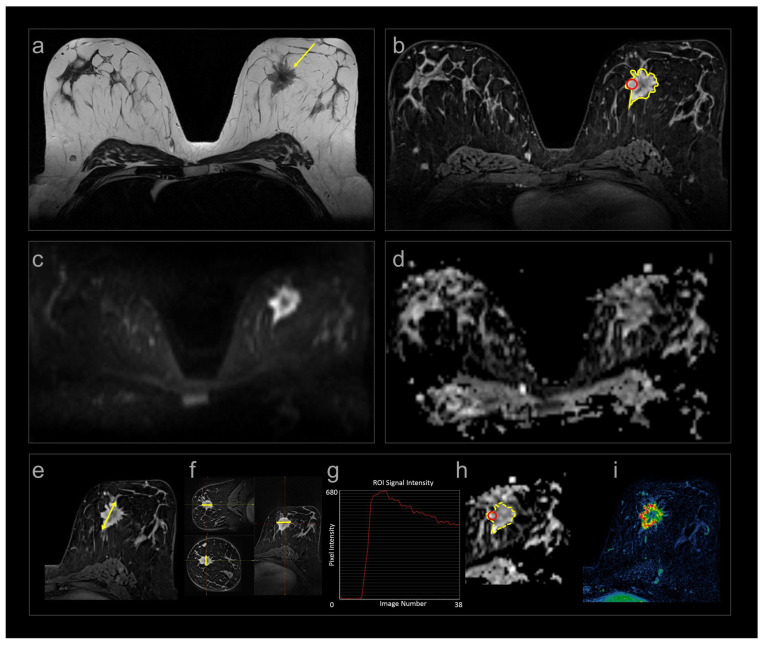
Multiparametric MRI (a–d) and measurements (e–i) in one patient at baseline. (a) Axial SE T2W image showing a spiculated tumour medially in the left breast (arrow); (b) corresponding DCE image with contrast enhancement in the tumour; (c) DWI b800; and (d) ADC maps of the same tumour. Treatment response was assessed by (e) longest diameter (yellow line); (f) calculated volume using the three perpendicular diameters (yellow lines) and the ellipsoid formula; (b) segmented volume (yellow delineation ); (g) signal intensity–time curves from focal area (red circle in (b)); (h) ADC values from focal area (red circle) and segmented volume (yellow delineation); and (i) quantitative volume transfer constant K^TRANS^.
